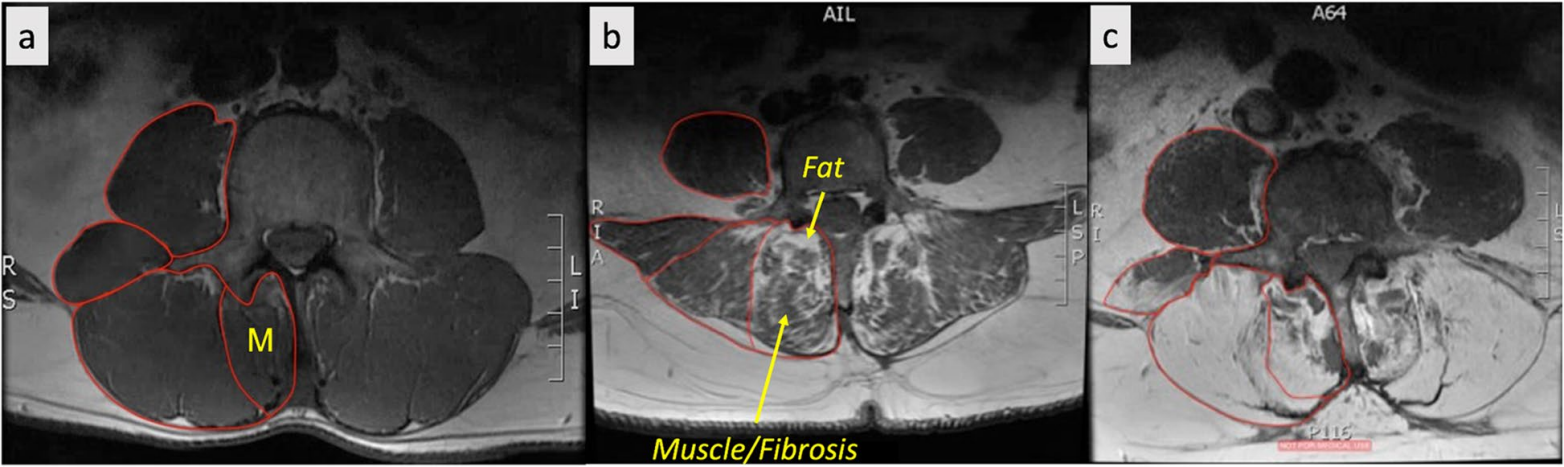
Pathological muscle adaptations. T2-weighted axial MRIs of the lumbar paraspinal muscles (red outlines) from three different individuals, depicting muscle degeneration. Panel (a) depicts an individual with minimal fatty infiltration of the multifidus (M) muscle compartment. Panel (b) depicts an individual with moderate fatty infiltration, while panel (c) depicts an individual with high fatty infiltration. In conventional T2-weighted MRI imaging, muscle and fibrosis both appear dark and fatty tissue appears bright, as depicted above
